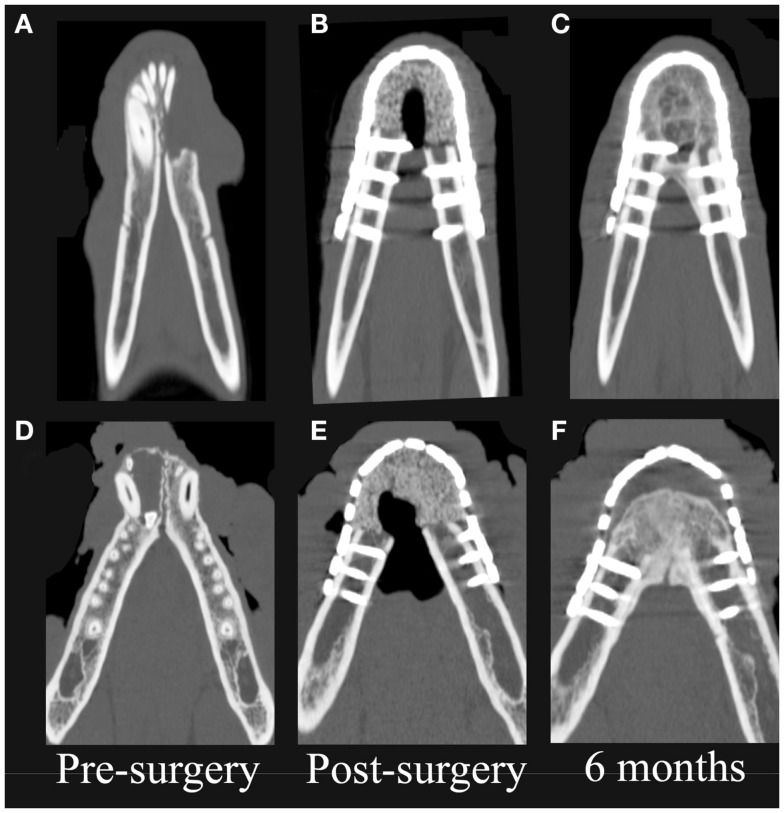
Dorsal-plane, reconstructed, CT images of the mandibles in two patients prior to surgery (A,D), immediately postoperatively (B,E), and 6 months after surgery (C,F). The right side of the patient is displayed at the left side of each image. Note geographic osteolysis of the rostral left mandibles in patient 1 (A) and of the rostral right mandibles in patient 2 (D) associated with squamous cell carcinoma and acanthomatous ameloblastoma, respectively. After 6 months, the borders between the scaffold and the native mandibles have become less distinct and new regenerated osseous tissue fills the rostral intermandibular space.
